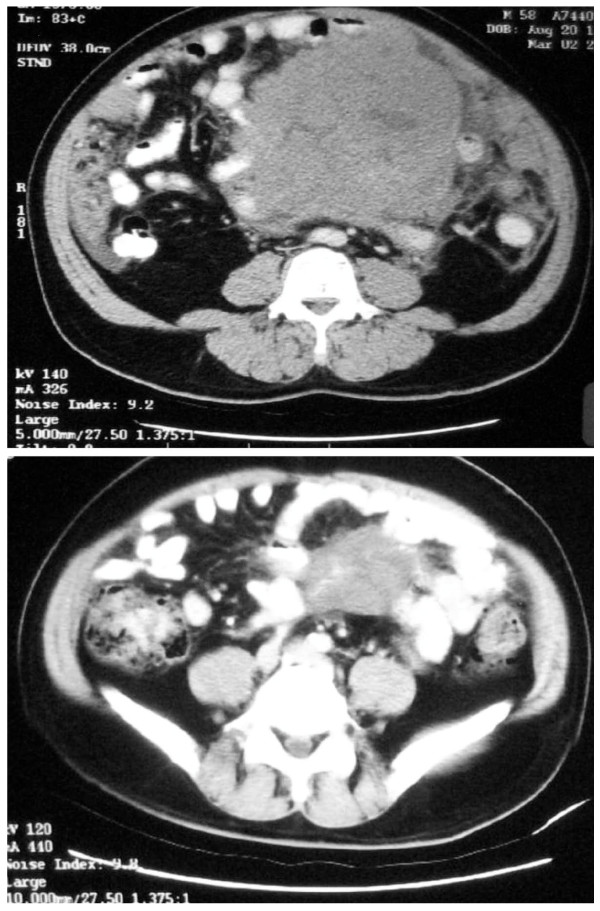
(A) CT abdomen showed a huge enhancing lobulated mass of 15.5 cm in diameter in the central abdomen before chemotherapy. (B) Reassessment CT abdomen after chemotherapy showed persistent central abdominal mass measured 7 cm in diameter.
